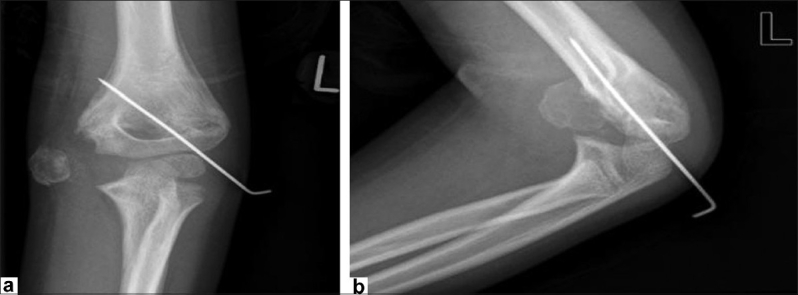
(a) Anteroposterior radiograph demonstrating a well circumscribed mass. (b) Lateral radiograph demonstrating a well circumscribed mass
